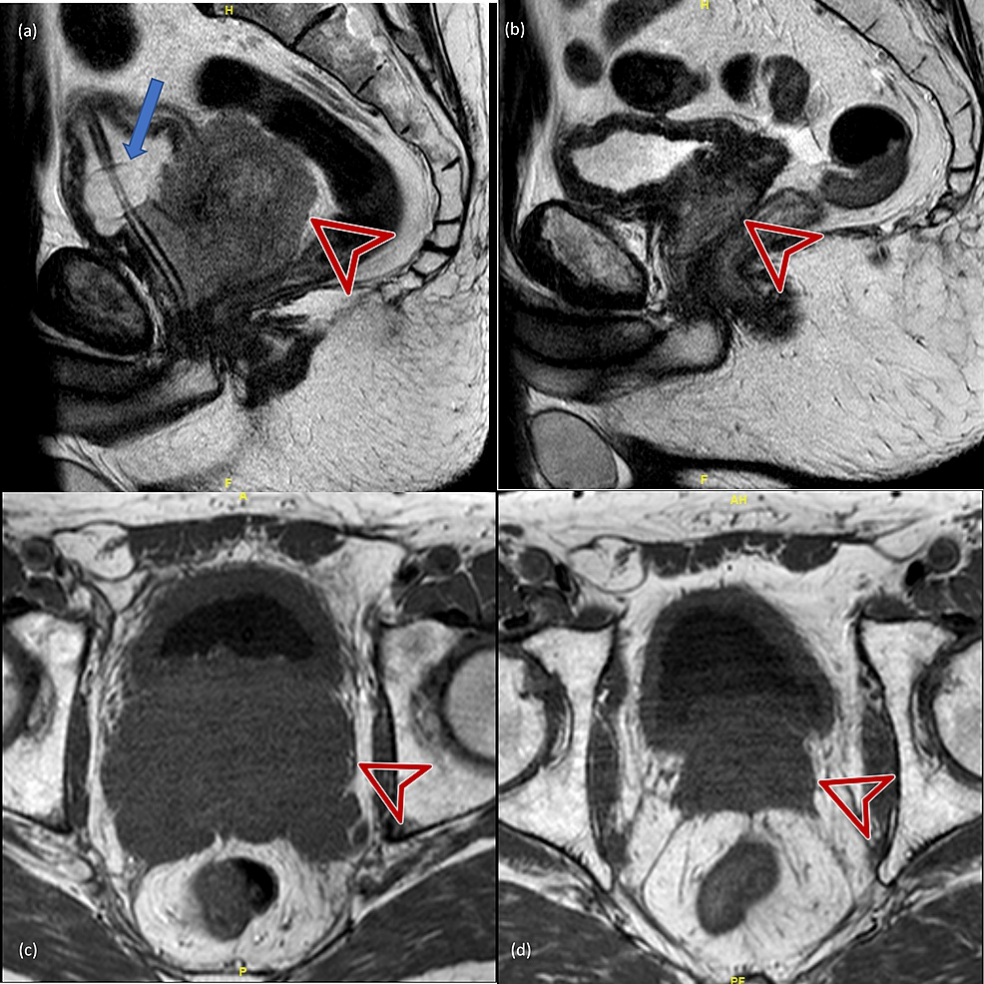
Pre-treatment vs. four-month post-treatment MRI scans. (a, c) T2 MRI scan pre-treatment showing a large heterogeneous prostate (red arrows). (b, d) T2 MRI showing a normal-appearing prostate (red arrows) four months after delivery of 50 Gy in 20 fractions. Top row = Sagittal view, bottom row = Axial view. The blue arrow points toward a Foley catheter.
